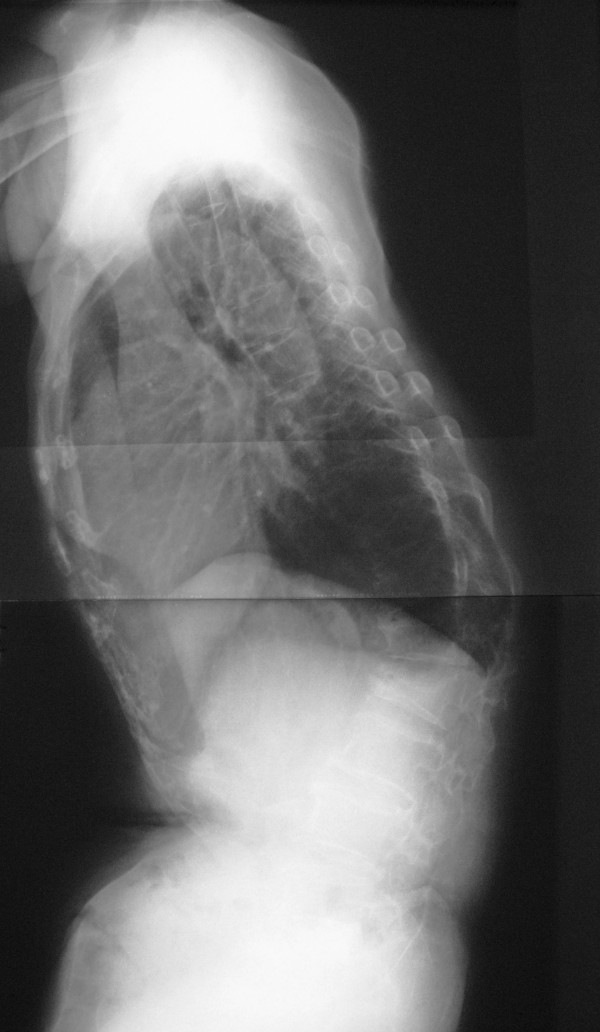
Preoperative standing lateral radiography of the spine showing osteoporosis and severe lower acute kyphosis (obvious thoracolumbar kyphosis with thoracic lordosis).
